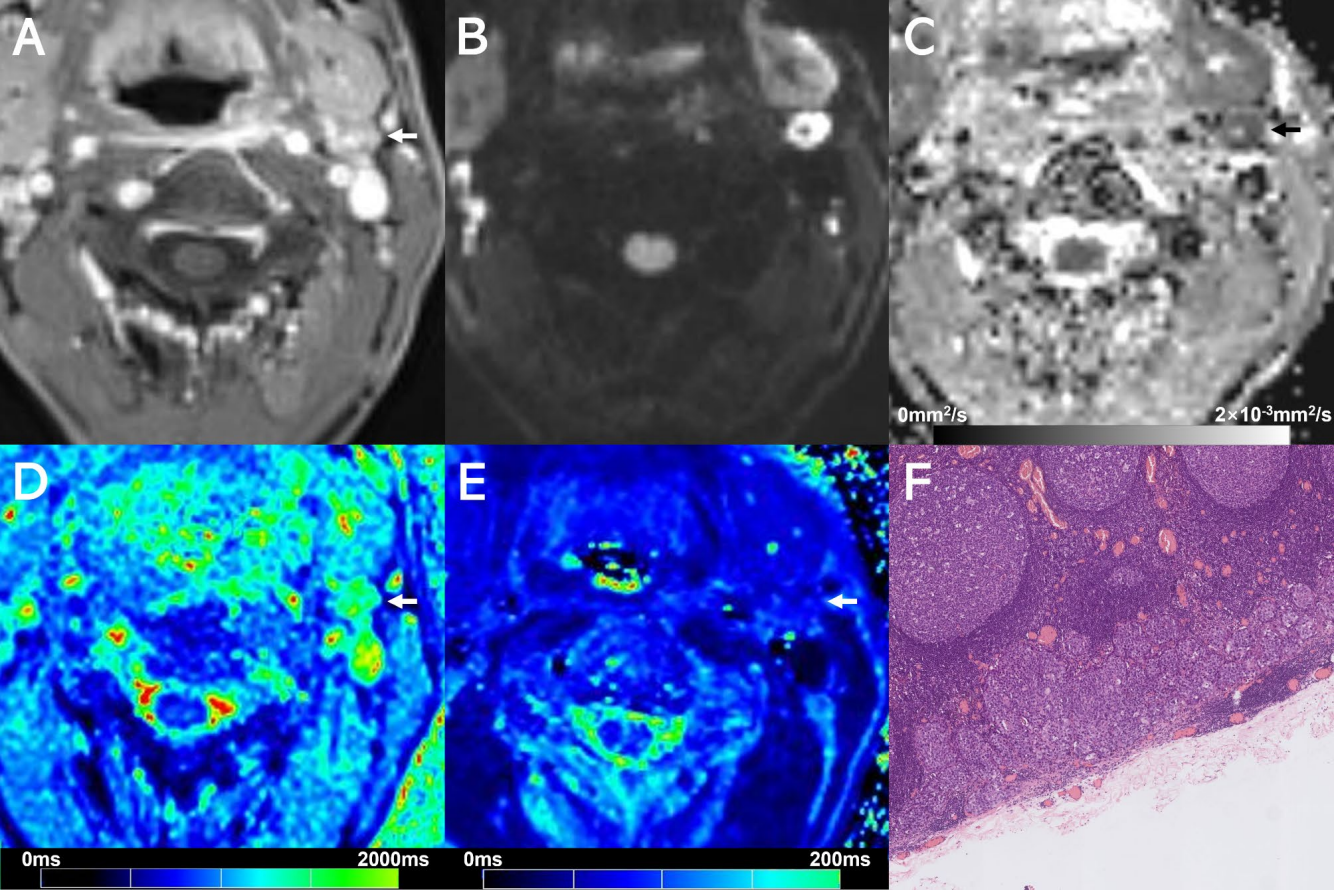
Images show a 7.5-mm size metastatic lymph node (arrows) at left level II from a 49-year-old male with hypopharyngeal squamous cell carcinoma. (A) Contrast-enhanced T1-weighted image shows the lymph node. (B) DWI with a b-value of 800 mm^2^/s shows a hyperintense area. (C) The ADC map on the targeted lymph node shows a hypointense signal, an ADC value of 0.831 × 10^− 3^ mm^2^/s. (D) T1 mapping and (E) T2 mapping show a T1 value of 1708.2 ± 216.6 ms and a T2 value of 76.6 ± 11.0 ms. (F) Section through the same lymph node showing metastatic deposit
